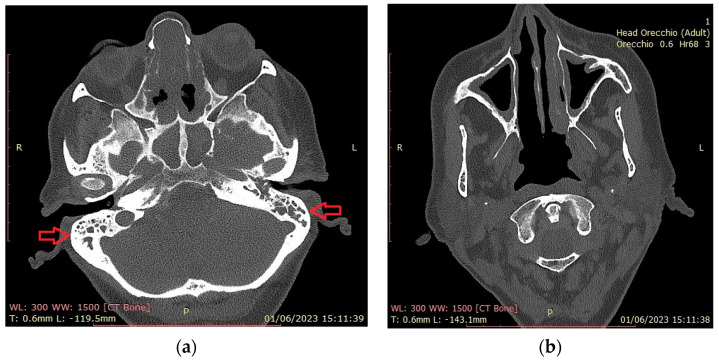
Head CT scan. (a) Total obliteration of the mastoid cells (red arrows) by a hyperdense phlogistic tissue. Total obstruction of sphenoidal and subtotal obstruction of etmoid sinuses; (b) hypertrophy of the maxillary sinus mucosa. Absence of the right inferior turbinate (asportation by previous surgery).
